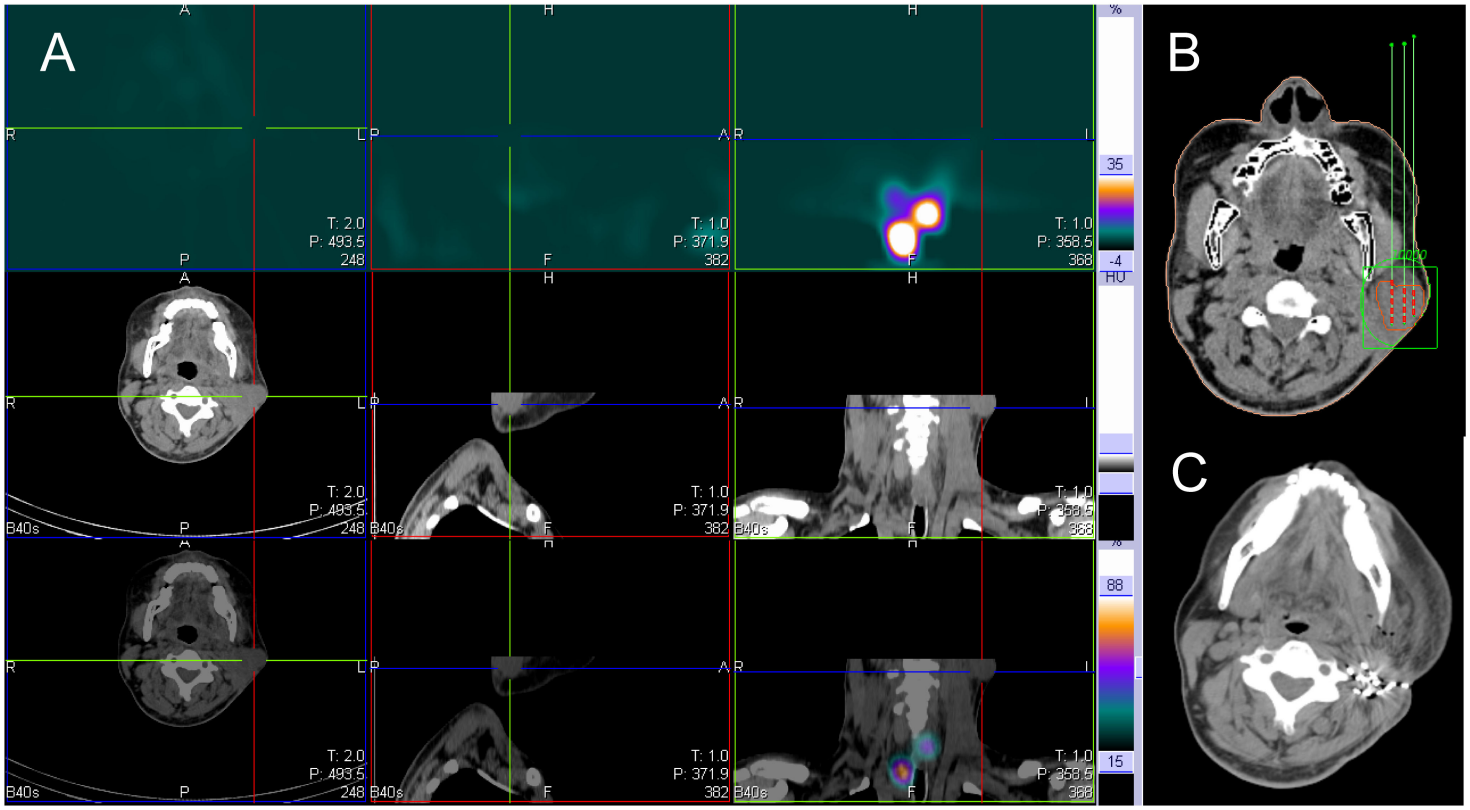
^131^I SPECT/CT imaging was performed on a 37-year-old male patient with ^131^I refractory differentiated thyroid cancer. The imaging revealed metastasis to the left neck lymph node, as indicated by the cross and arrow (shown in the image). (A) The ^131^I SPECT/CT neck fusion imaging showed no iodine uptake in the left cervical lymph node metastases. (B) The TPS was used for preoperative needle placement. (C) After 6 months, reexamination of the left cervical lymph node showed significant shrinkage, particle aggregation. SPECT/CT, Single Photon Emission Computed Tomography/Computed Tomography; TPS, Treatment planning system.
